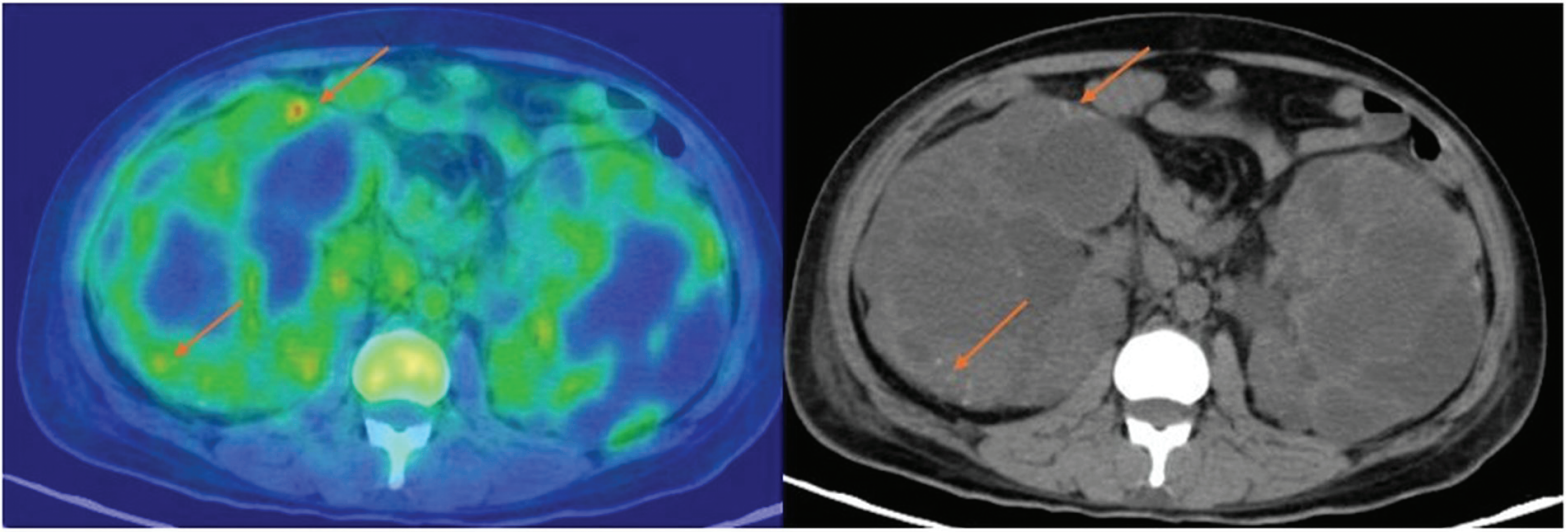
A 34-year-old gentleman with autosomal dominant polycystic kidney disease (ADPKD) and persistent fever, a Ga-68 citrate positron emission tomography/computed tomography (PET/CT) scan was performed to rule out infection or hemorrhage, Ga-68 citrate avidity was noted in areas corresponding to hyperdense cysts in both the kidneys and was further advised for ultrasonography (USG)-guided aspiration from the target lesions for further confirmation, which was performed and confirmed as hemorrhage with no infective growth in the culture. This was regarded as a false positive finding.
